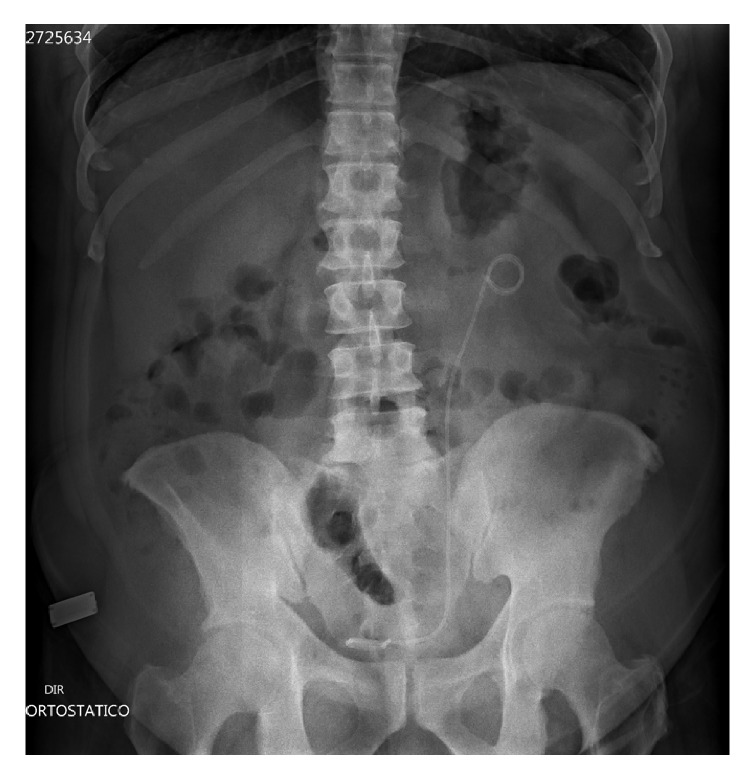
Abdominal X-ray showing absence of residual calculi in left pyelocalicial system.
